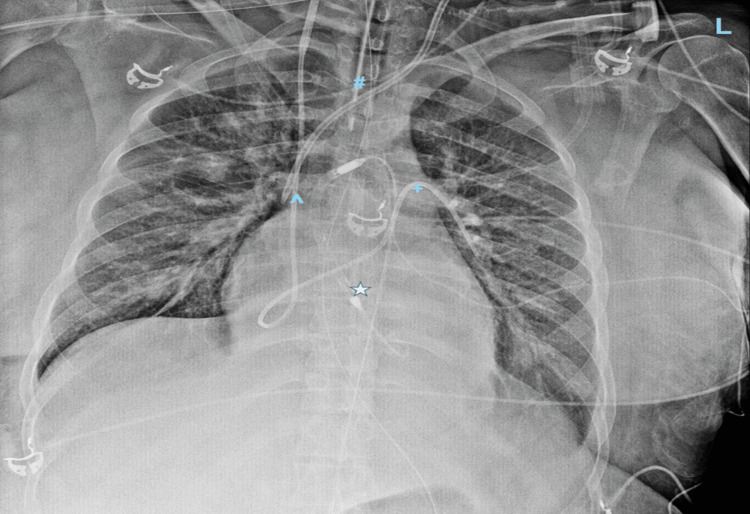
Antero-posterior chest x-ray demonstrating Impella CP device placement in LV cavity *Impella CP via femoral approach, ^#^endotracheal tube, ^central venous line, and ^+^pulmonary artery catheter with an enlarged cardiac silhouette and pulmonary congestion. LV, left ventricular
